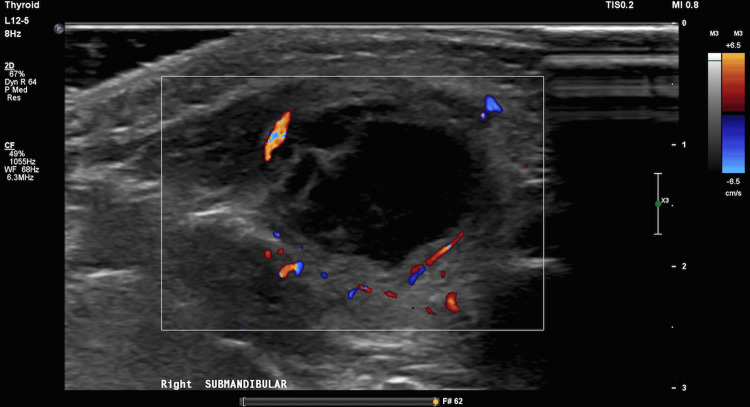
Doppler ultrasound submandibular view of the right parotid abscess
